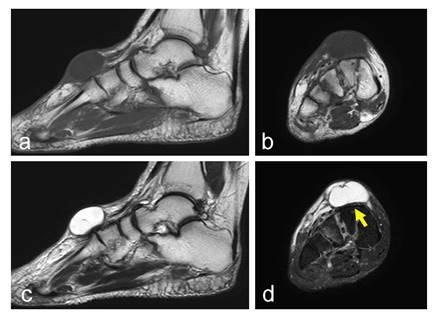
A 66-year-old female with a ganglion in the dorsal foot. The lesion, with low signal intensity on a T1-weighted image (a–b) and high signal intensity on a T2-weighted image (c–d), is shallowly located over the cuneiform. The dorsalis pedis artery is located below the lesion (yellow arrows; d) A T2-weighted image with fat-suppression (d). Sagittal views (a, c) and axial views (b, d).
